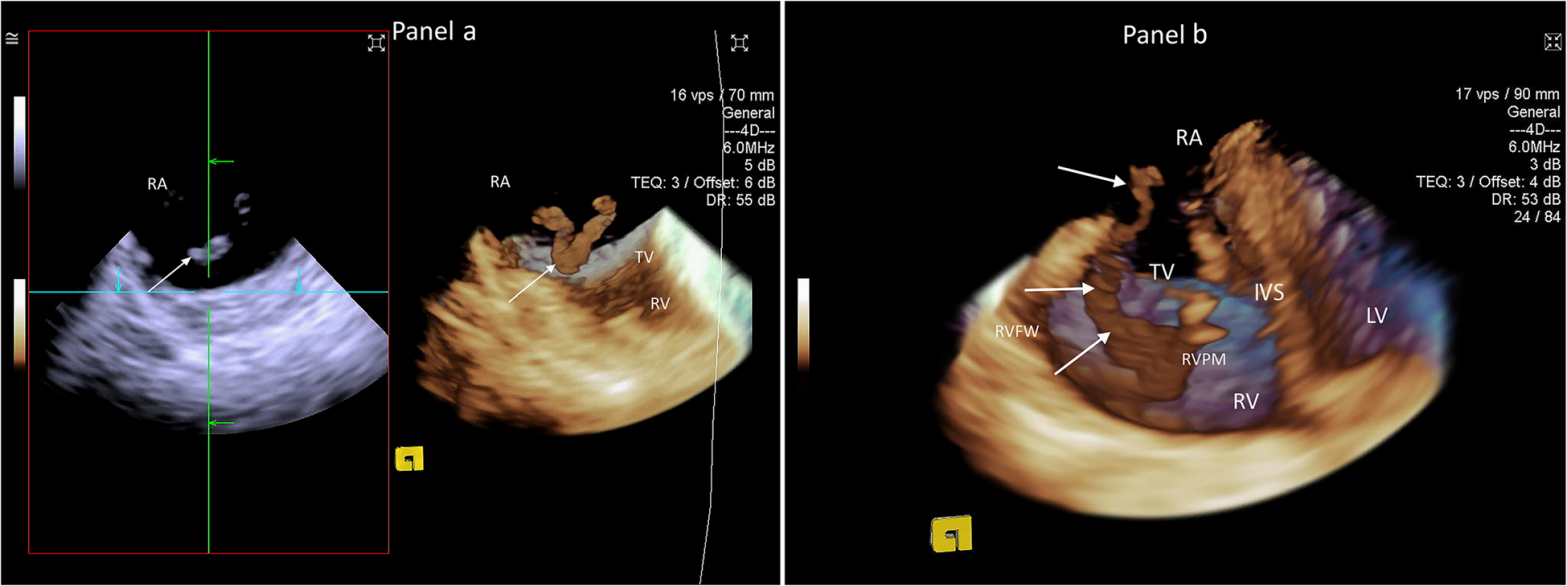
Right atrial and right ventricular clot-in-transit demonstrated with 3D ICE. Panel a: Right atrial mobile clot (white arrow) in two-dimensional and three-dimensional intracardiac echocardiography imaging. 3D ICE offers significantly better spatial appreciation of the clot size and position inside cardiac chamber. RA indicates right atrium; TV, tricuspid valve; RV – right ventricle. Panel b: Large mobile clot (white arrows) is clearly demonstrated in three-dimensional intracardiac echocardiographic image within right atrial and right ventricular cavities. It is prolapsing through the tricuspid valve towards right ventricular apex. The right ventricle is dilated with the interventricular septum bowing to the left. RA indicates right atrium; RV, right ventricle; TV, tricuspid valve; LV, left ventricle; RVFW, right ventricular free wall; RVPM, right ventricular papillary muscle
